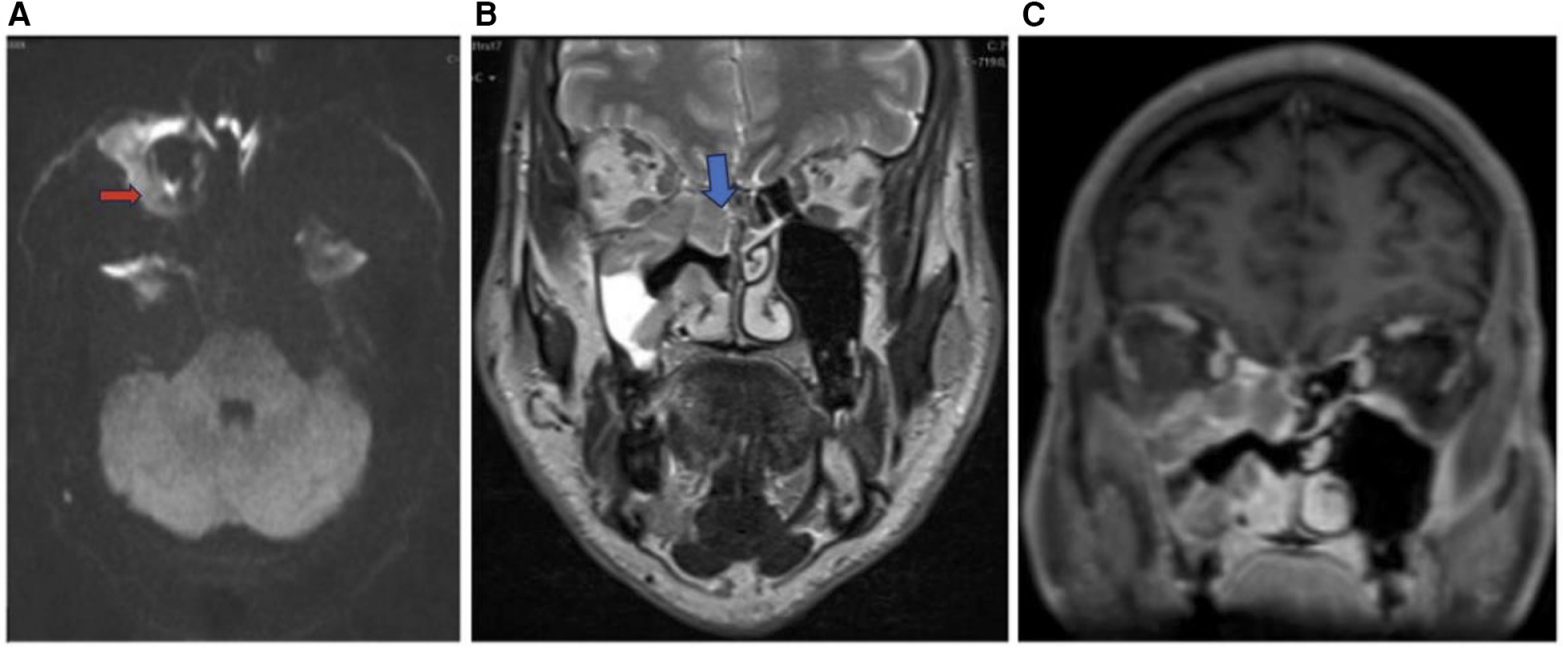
Sinonasal lymphoma. Axial DWI (A), coronal T2 (B), and coronal T1 + C (C) images showing sinonasal lymphoma centered in the right maxillary sinus (red arrow) extending to the right ethmoid (blue arrow) and right inferior orbital margin. Pathology confirmed CD5 and CD10 positive germinal center B cell DLBCL.
